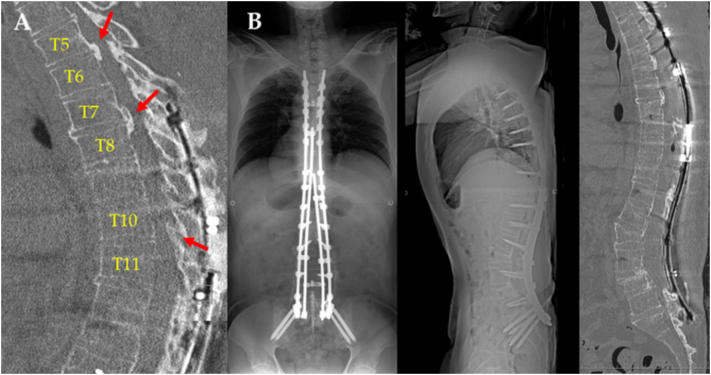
A) CT scan on postoperative day 5, just after onset of neurological symptoms, showing OPLL and OYL at T5/6 and T7/8, and OYL at T10/11 (arrows). B) Extensive laminectomy and extended fixation up to T3 were finally performed.
